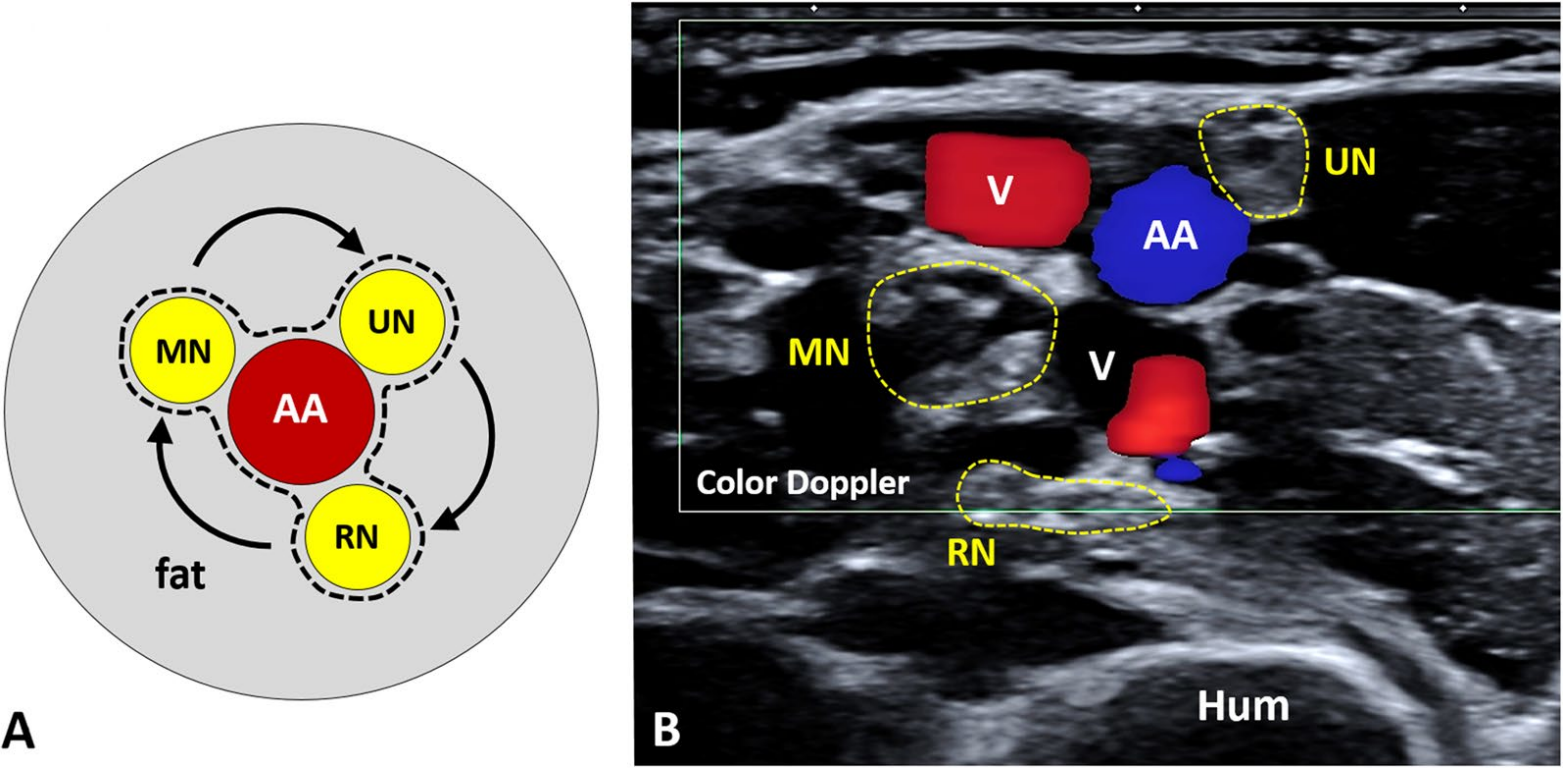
Neurovascular bundle of the axilla. The ulnar nerve *(UN)*, the radial nerve *(RN)* and the median nerve *(MN)* wrap the axillary artery *(AA)* in a clockwise fashion *(curved dark arrow)* at the axillary base (A). They present a typical honeycomb pattern *(yellow dotted line)* and can be easily differentiated from the vascular elements using the color/power Doppler (B). V: vein; Hum: humerus; black dotted line: axillary sheath
